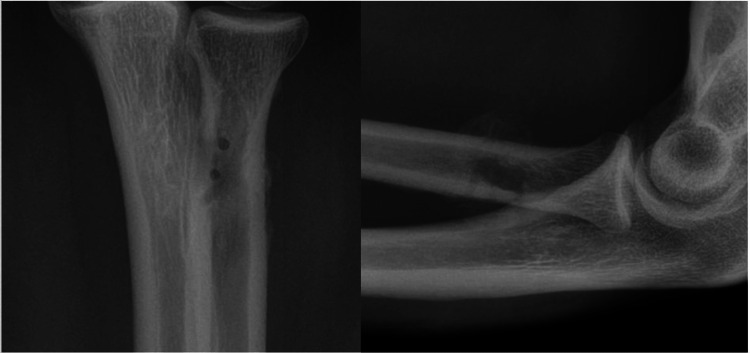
Anteroposterior and posterior X-ray view (author's original image) Spontaneous heterotopic ossification resorption at 24 weeks postoperatively.
